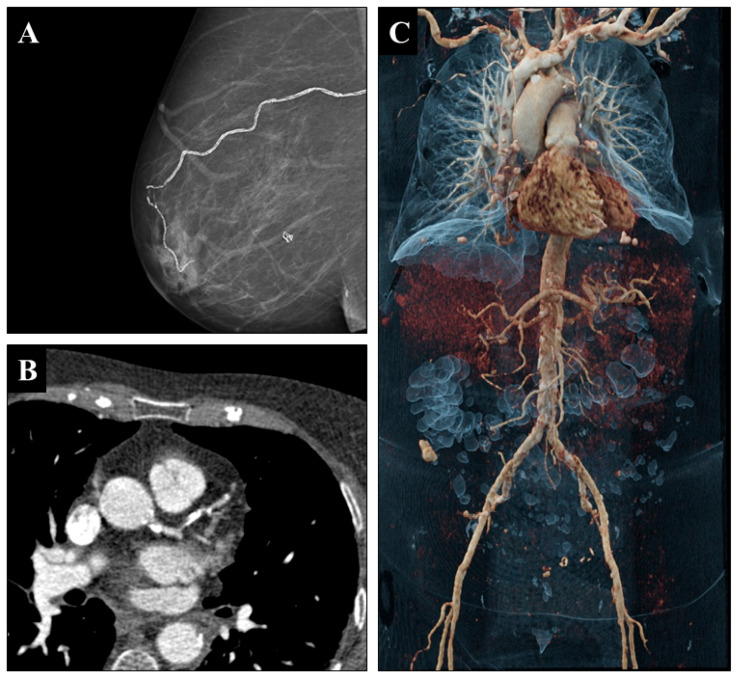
Screening mammography in a female patient in her late fifties, performed approximately eight years after bilateral lung transplantation, demonstrates severe breast arterial calcifications in the right breast on the MLO projection Image (A). Coronary CT angiography performed about 2 years later shows severe calcifications in left anterior descending artery and the left circumflex artery Image (B). A three-dimensional cinematic rendering of a CT scan performed in the same year depicts the aorta and major branches with advanced systemic vascular calcifications Image (C).
